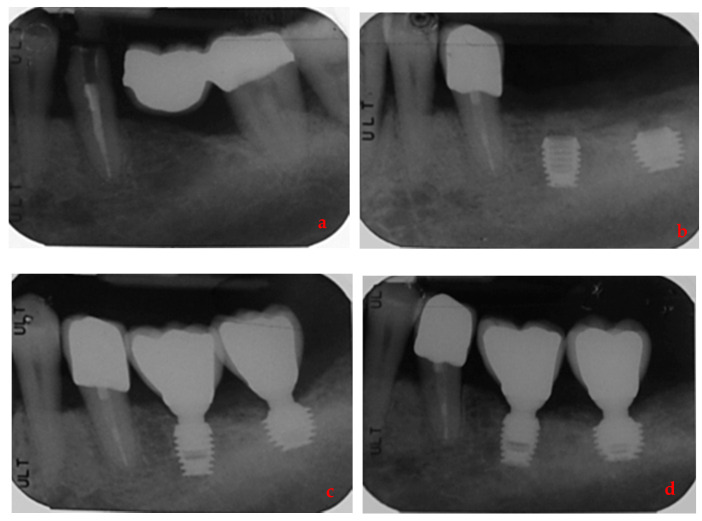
Single implants placed in 3.6 and 3.7 sites (4.5 × 6 mm and 6 × 5 mm) of a male patient with history of periodontal disease: (a) pre-operative radiograph before implants placement; (b) radiograph obtained at implants placement; (c) radiograph obtained at time of loading; (d) radiograph obtained at 3-year follow-up.
